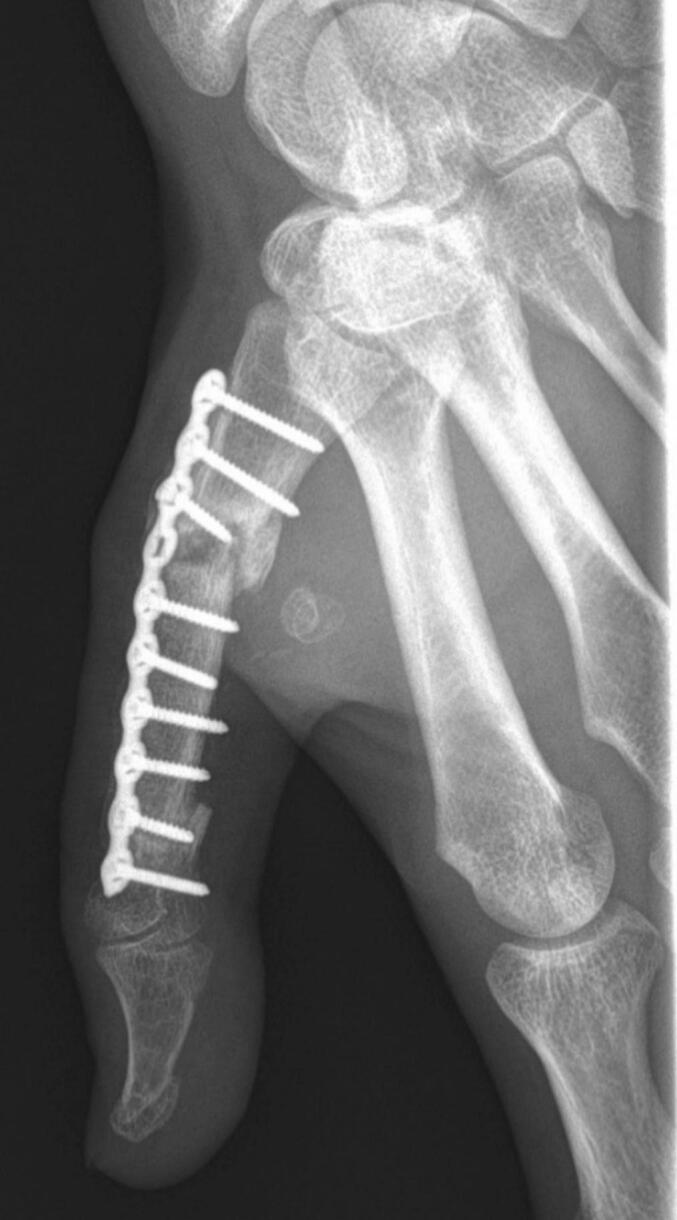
Frontal radiograph at 12 months postoperatively, demonstrating bone consolidation with ossification.
